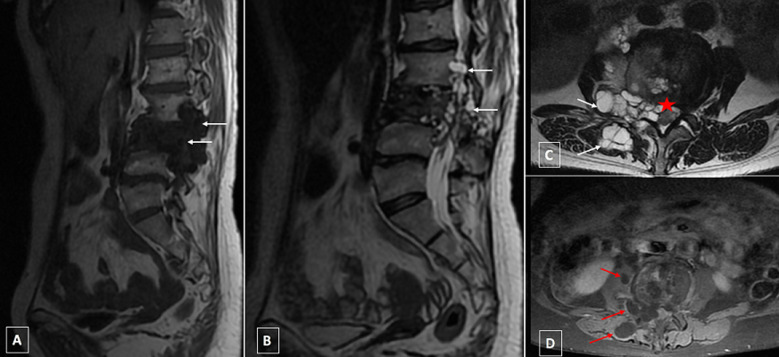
lumbar MRI in sagittal (A,B) and axial sections (C,D) respectively: A (T1WI), B and C (T2WI), D (post gadoliniuim injection) showing multiple cysts (white arrows) at L3 level presenting low signal on SE T1 WI (A) and high signal on SE T2 WI (B) non enhanced after gadolinium injection (D), with extension into the spinal canal and compression of the cauda equina (star) and into the peri vertebral soft tissue (red arrow): dumbbell formation according to Braithwaite and Lees classification
